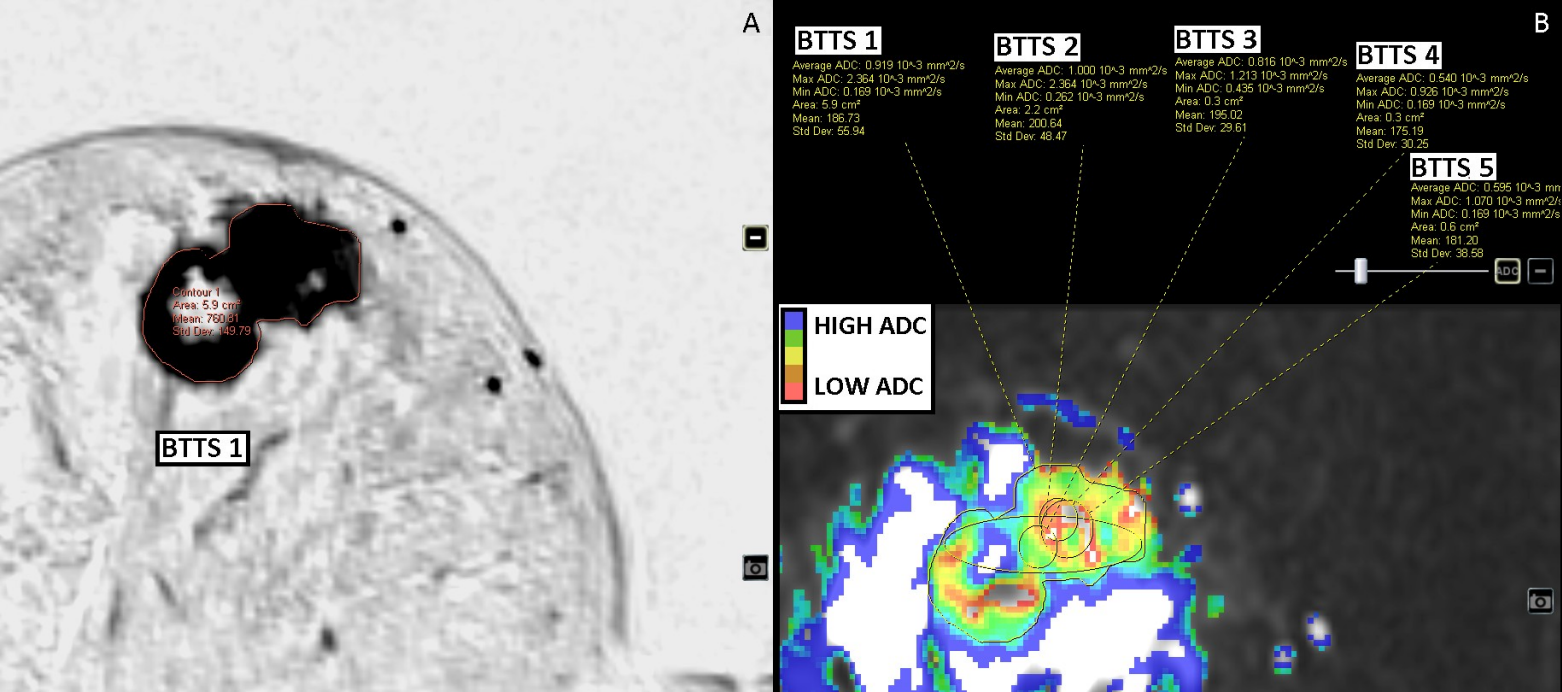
Invasive ductal carcinoma of the right breast, ER and PR positive, HER2-neu negative. DCE-T1 subtracted images (inverted) (A) and DWI b0-1000 images (B).
